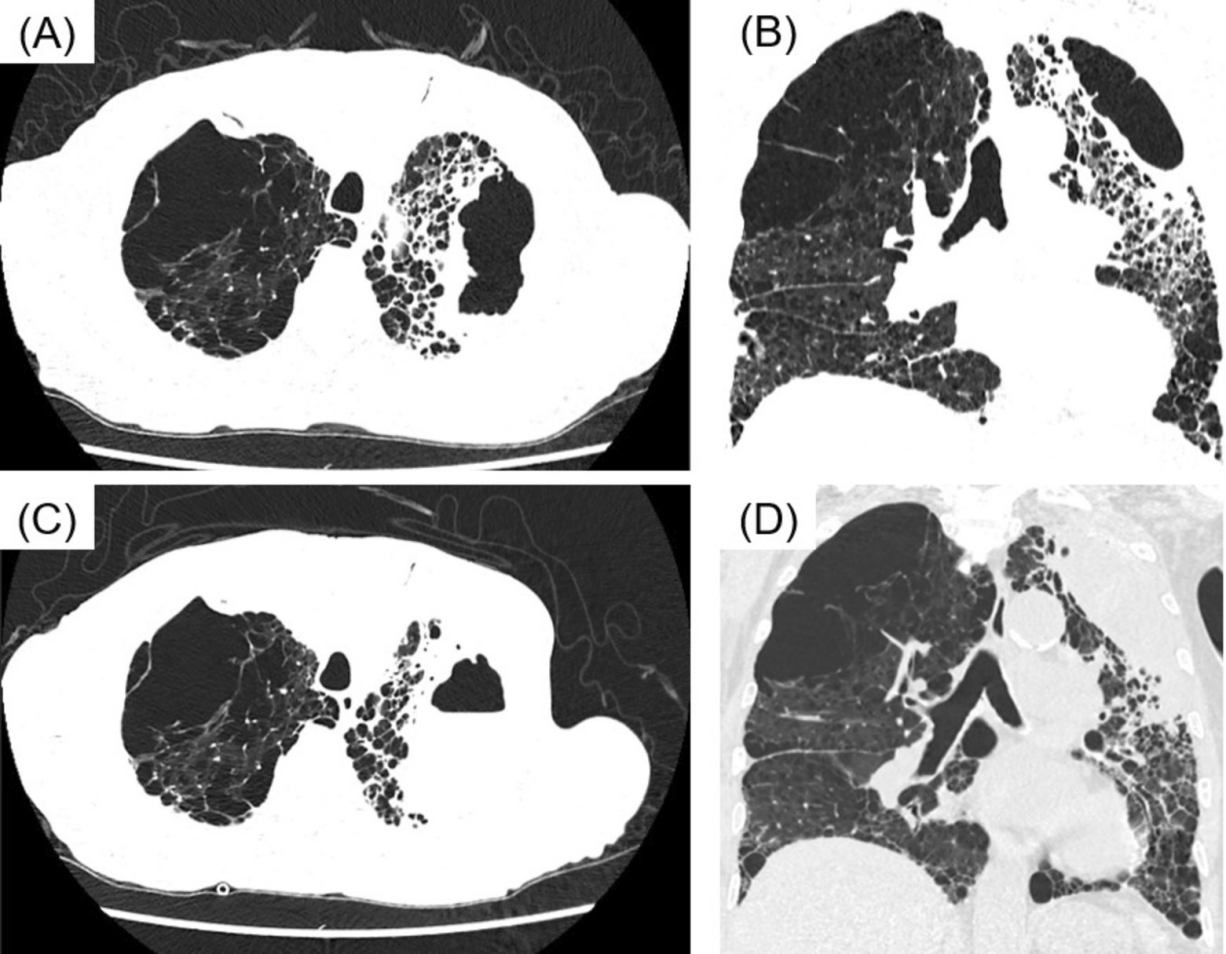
Chest computed tomography images. (A) Axial chest computed tomography image on admission. (B) Coronal chest computed tomography image on admission. (C) Axial chest computed tomography image on hospital Day 15. (D) Coronal chest computed tomography image on hospital Day 15.
